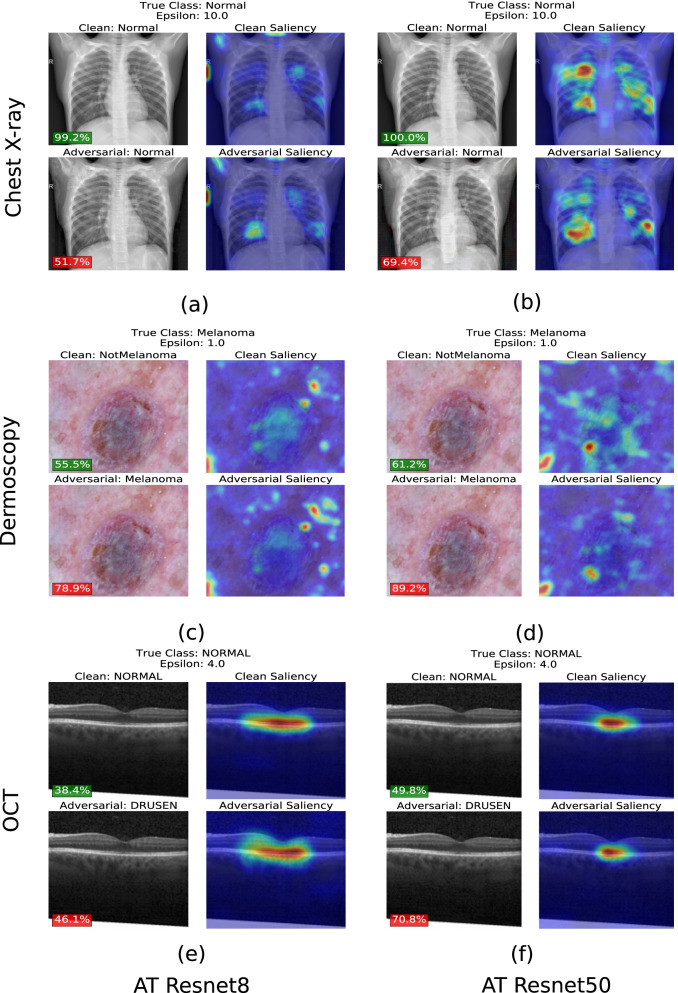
Saliency maps of adversarially trained (AT) Resnet8 (a, c, e) and Resnet50 (b, d, f) for Chest X-ray, Dermoscopy & OCT datasets. Unperturbed images with predicted labels and corresponding saliency maps were visualized on row 1 (no attack) for (a–f), respectively. Imperceptibly perturbed images with predicted labels and corresponding saliency maps were visualized on row 2 (PGD attack) for (a–f), respectively. Saliency maps of Resnet50 are more concentrated on the regions of interest whereas Resnet8 include attention regions that are more sporadic on areas that do not contribute to the classification of the disease
